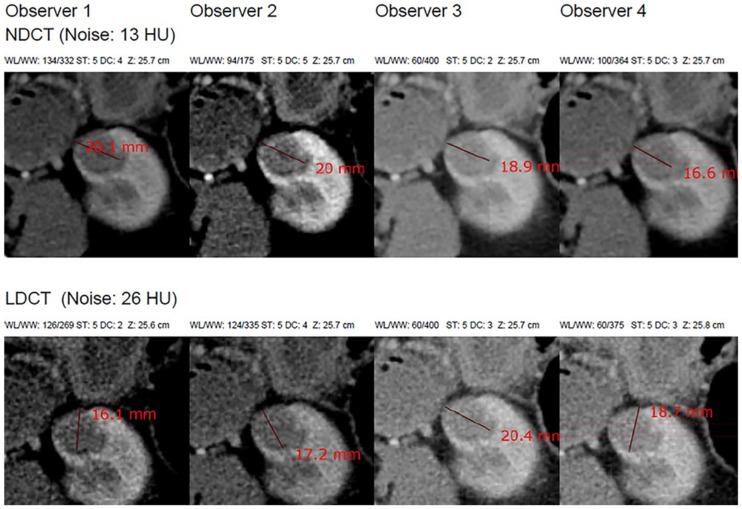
Observer-specific renal tumor diameter measurements from normal-dose CT (NDCT) and low-dose CT (LDCT) for Case 1 show that, despite LDCT having higher image noise, diameter estimates remain consistent across observers and dose levels [13].
